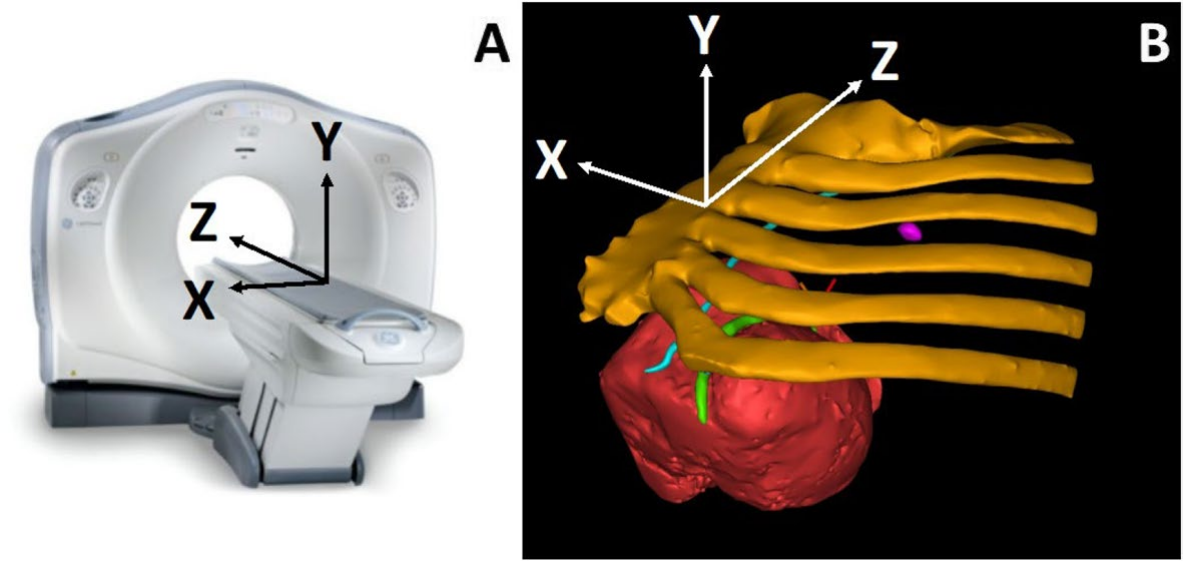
Cartesian coordinate system for distance measurements made from anatomic volumes. A The bed of the CT scanner and the OR are considered identical for the measurements with the positive “Z-axis” defined as pointing towards the patient’s head. The X and Y axis were then determined by the right-hand rule for spatial relationships by respect to the CT scanner or the operating room table and (B) along the orientation of the anatomic parts segmented for 3DP. Volume rendering after image segmentation shows the same orientation of the cartesian coordinates. Since all the measurements are differences, a specific definition of the origin (x, y, z = 0, 0, 0) was not necessary
